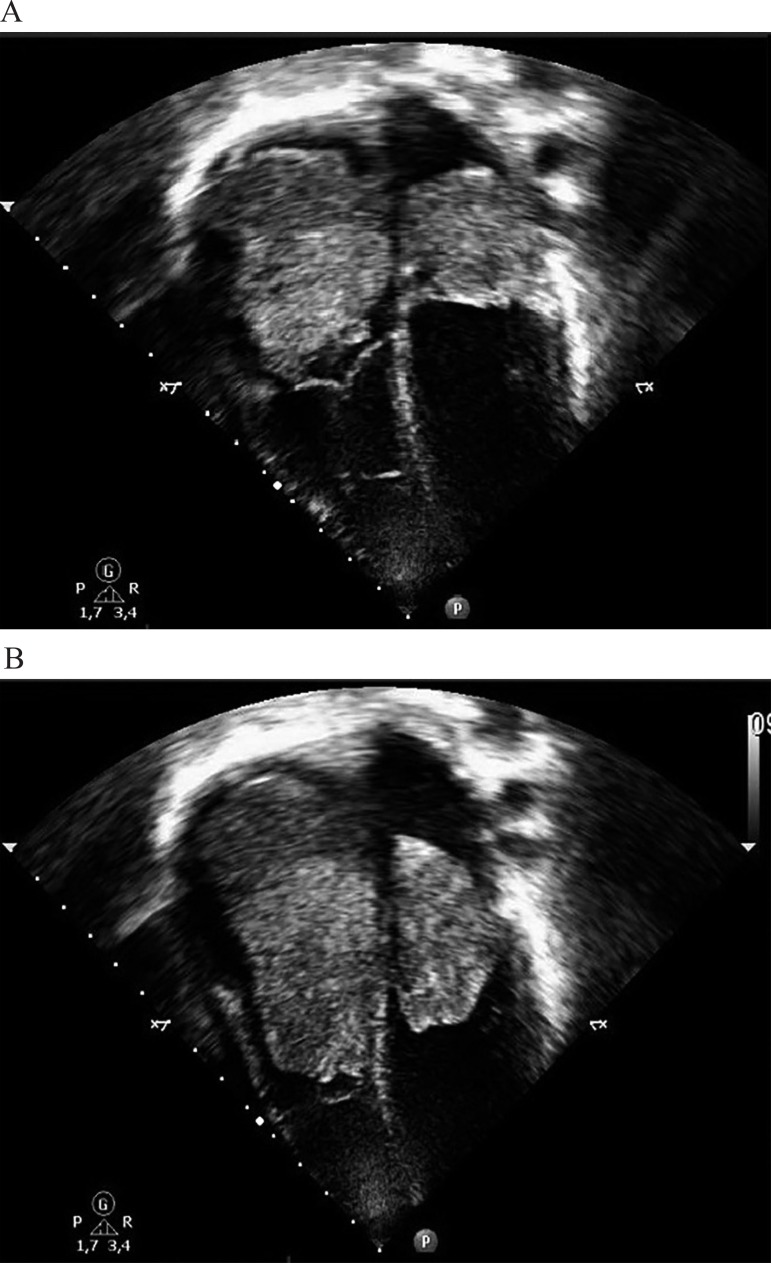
A. Two-dimensional echocardiogram preoperatively showing the masses within
